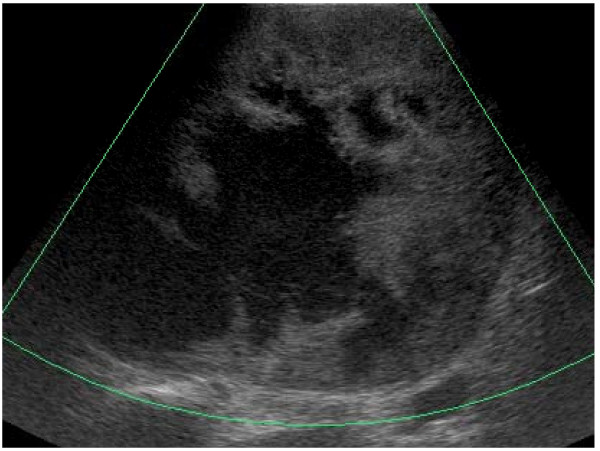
Abdominal ultrasonography revealed an 18 × 18 cm, heterogeneously hypo-echoic lesion in the right adrenal area with peripheral high-echoic rim.
